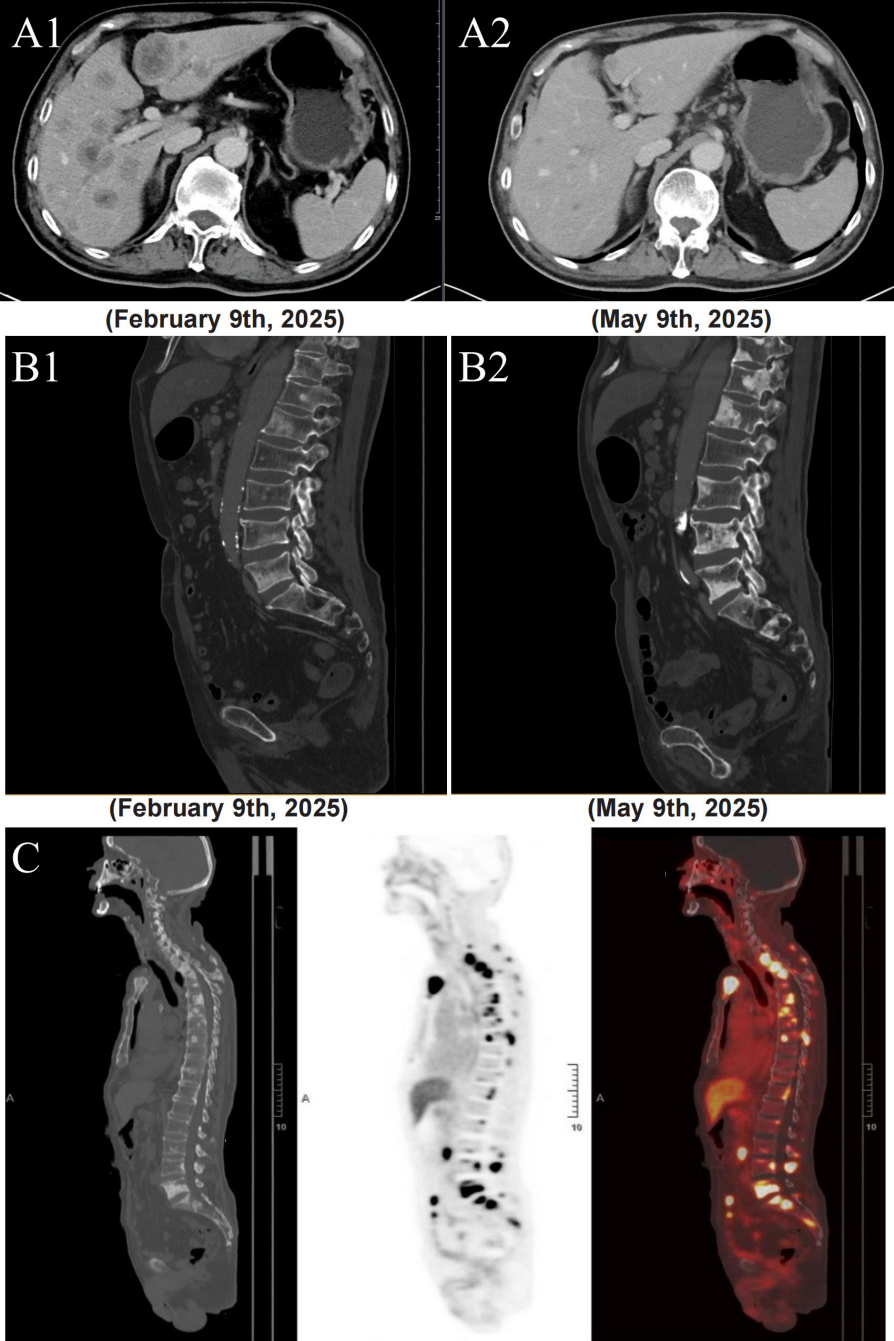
(A1, B1) Multiple metastatic tumors in the liver and bone shown in the enhanced abdominal CT scan. Before the treatment with Disitamab Vedotin. (A2, B2) After three treatments with 120 milligrams of Disitamab Vedotin. (C)^18^F-PSMA PET/CT demonstrating radiotracer uptake in liver (SUV max=7.52) and bone (SUV max=14.33).
